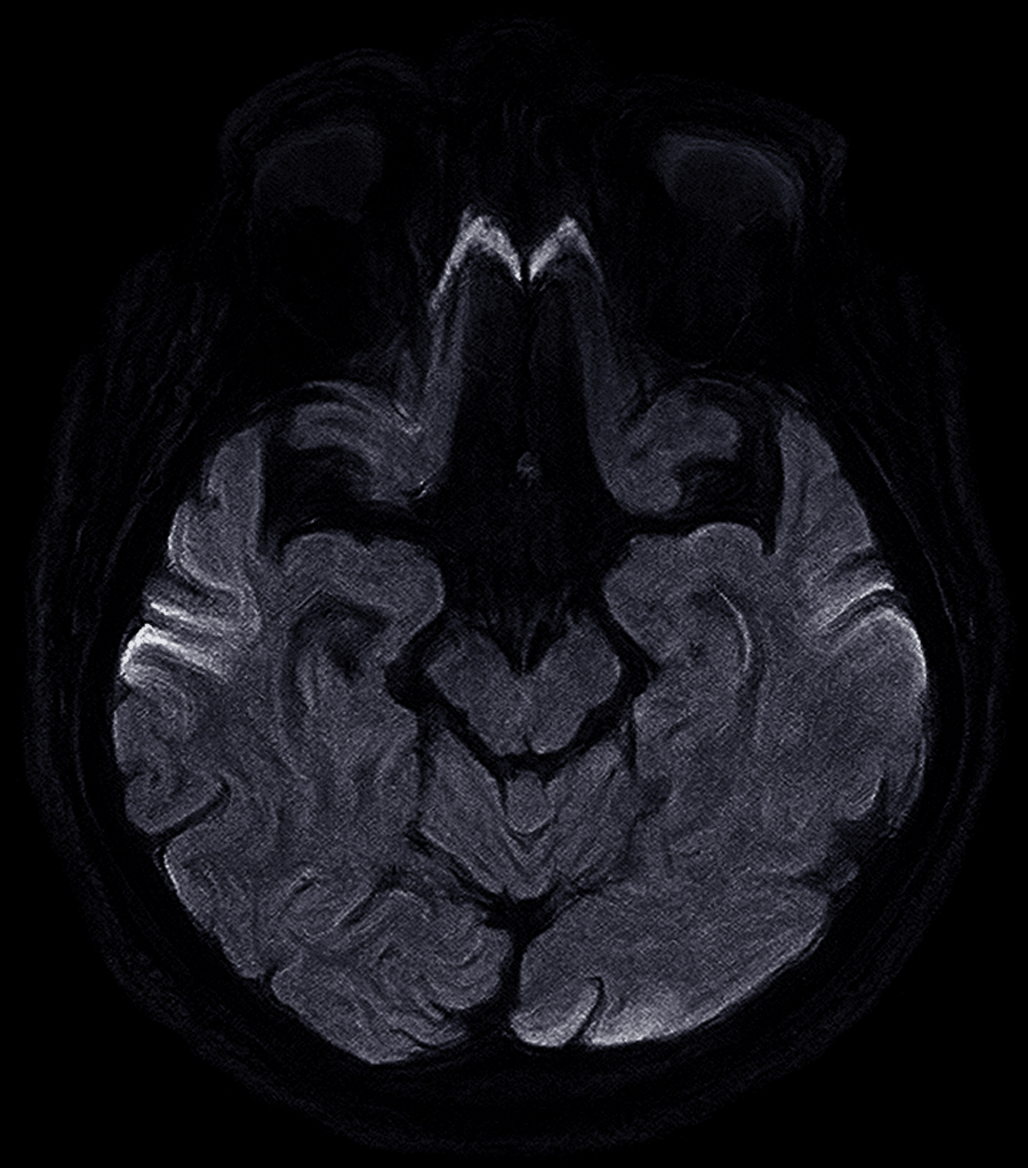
MRI brain ​​​​​​​diffusion-weighted imaging (DWI) and FLAIR sequence shows cortical FLAIR hyperintensities and diffusion restriction involving the bilateral parietal lobes. FLAIR: fluid-attenuated inversion recovery
